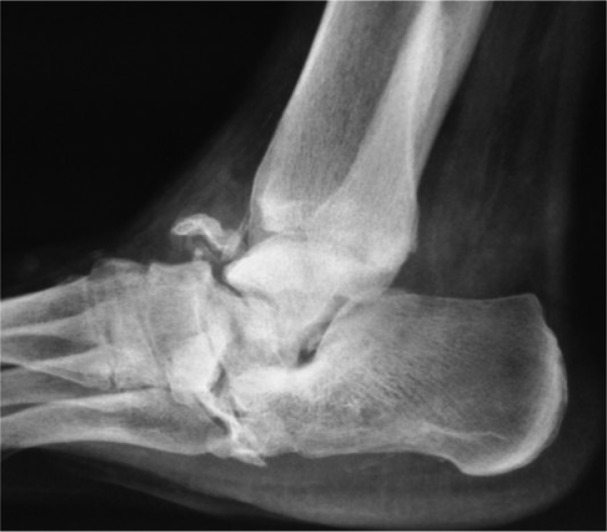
Plain radiographic lateral ankle view showing a collapse of the talar head and neck with resorption of the talus.
